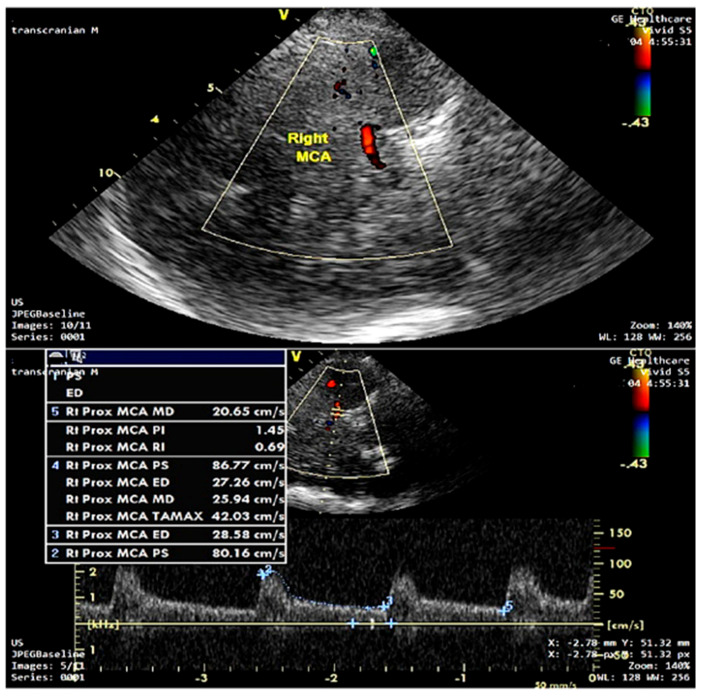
Transcranial Doppler ultrasound recording of the middle right cerebral artery (MCA). The spectrum shows peak systolic velocity (PSV), end-diastolic velocity (EDV), and mean blood flow velocity (MBFV) used for the calculation of the pulsatility index (PI) and resistivity index (RI).
